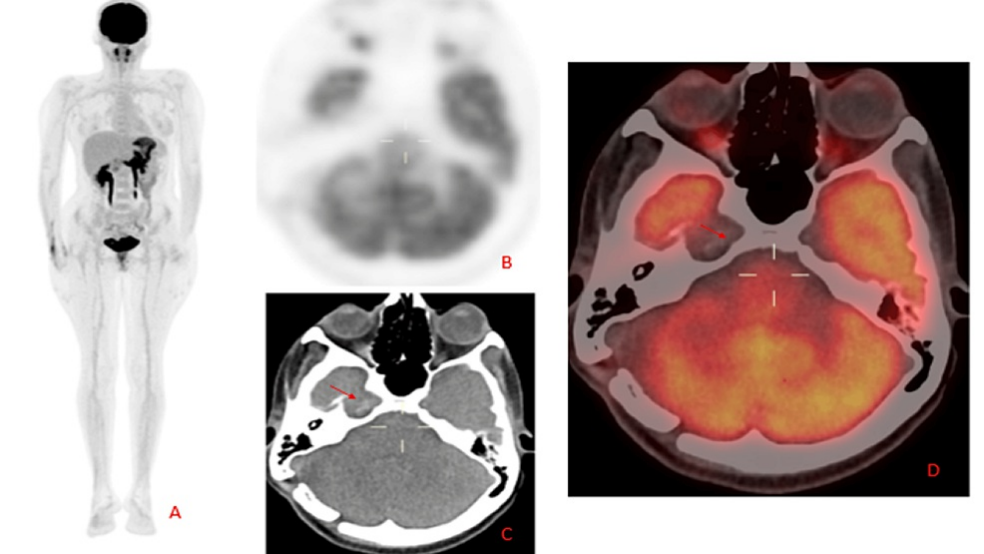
PET scan showing no evidence of distant metastasis (image A). Hyperdense area on PET CT image B and (red arrow in) image C within the right Meckel's with no significant increase FDG uptake (red arrow in image D) FDG: Fluorodeoxyglucose
